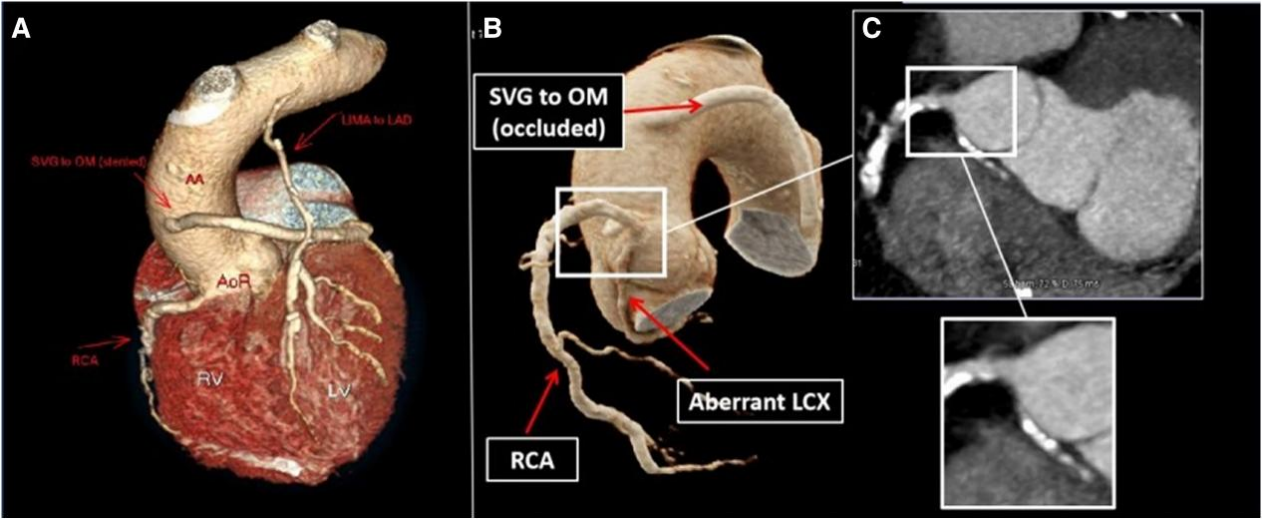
(*A*) Three-dimensional (3D) reconstructions of the patient’s native coronary arteries and bypass grafts are depicted using computed tomography coronary angiogram (CTCA). (*B*) 3D reconstruction demonstrating anomalous left circumflex (LCX) originates from the anterior/inferior position off the right coronary sinus of Valsalva, with an acute posterior-lateral angulation. (*C*) Two-dimensional (2D) coronal cross-section image showing severely calcified proximal anomalous LCX with an extremely inferior take-off from the right coronary sinus of Valsalva, 6 mm inferiorly to the right coronary artery (RCA) ostium.
